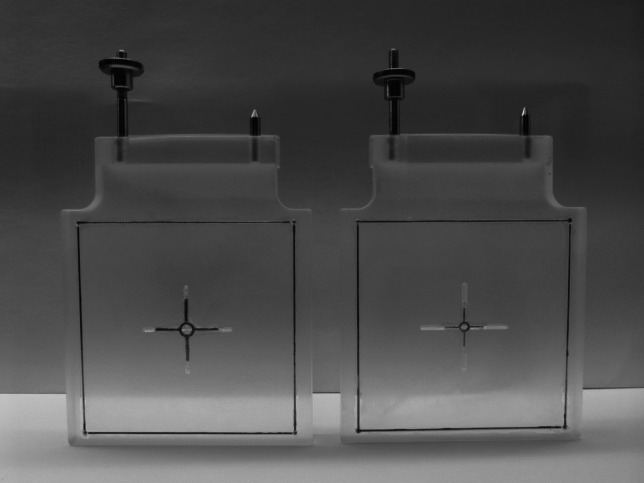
The angio-plates used in conventional fluoroscopic procedures are modified by adding a ring and a cross to the center of each plate. The dimensions of the markers on every two opposing plates respect the different magnification factors of each plate. The plate closer to the source of the x-ray beam has smaller markers (relatively significant magnification), while the other plate (left) is marked with larger ring and cross (relatively minimal magnification)
